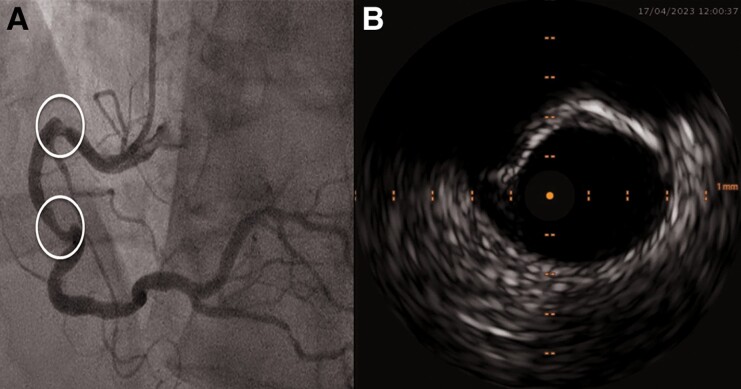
(*A*) Index right coronary artery lesion angiography. (*B*) Intravascular ultrasound of the proximal right coronary artery stenosis.
